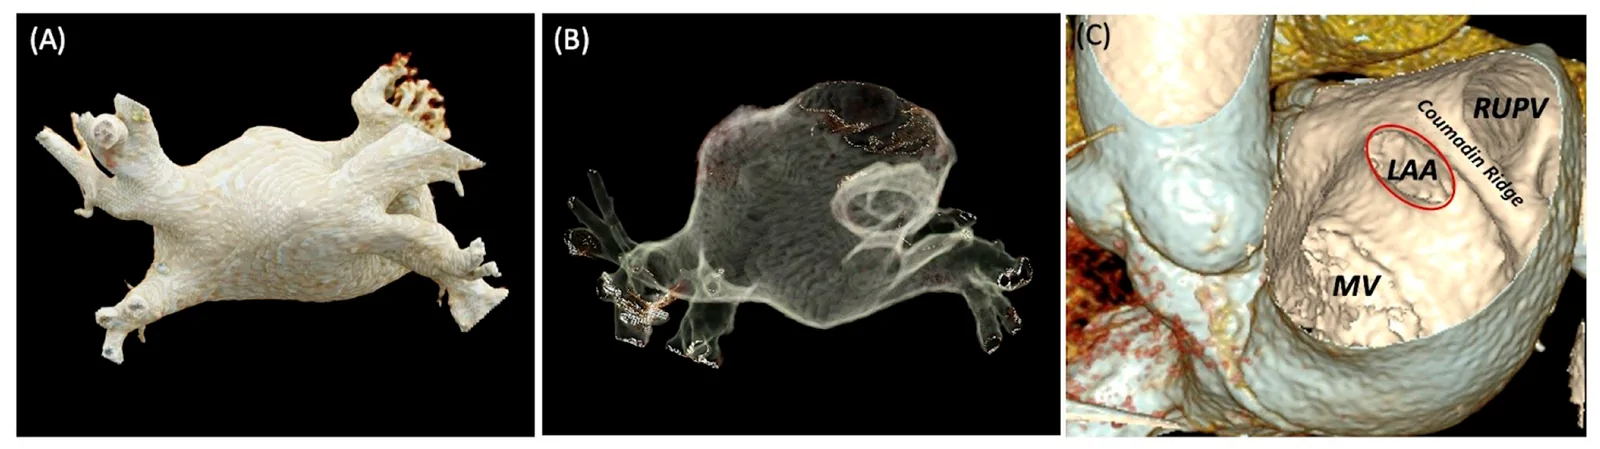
Advanced CT assessment of the LAA: 3D cinematic rendering (panel (A)) and translucent reconstruction (panel (B)) easily depict anatomy of the left atrium and pulmonary veins; intracavitary reconstruction better defines the relationship of the LAA ostium with surrounding structures (panel (C)). MV = mitral valve; LAA = left atrial appendage; RUPV = right upper pulmonary vein.
